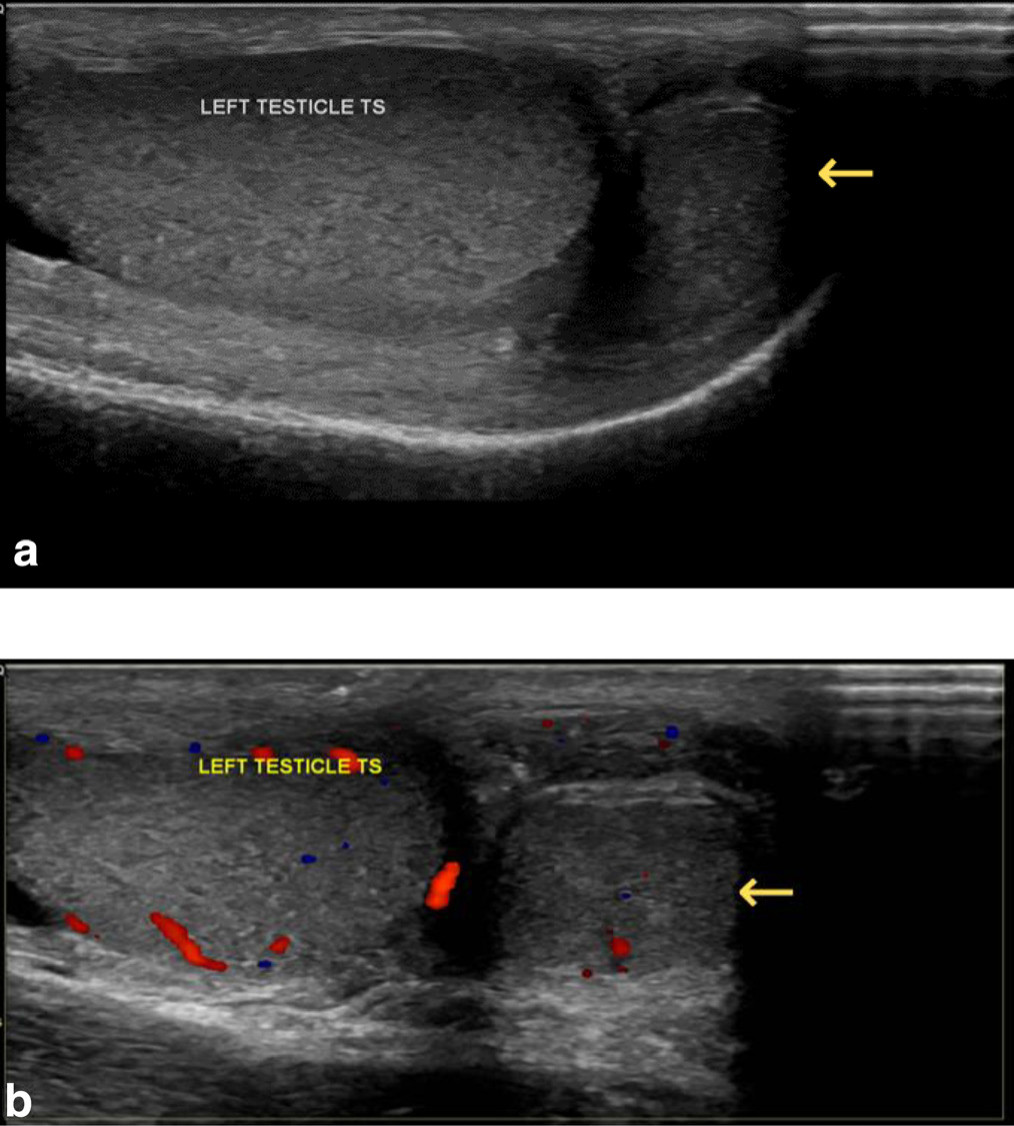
Longitudinal US image (A) and colour doppler US image (B) of the left testis showing a soft tissue mass adjacent to the left testis with similar sonographic appearances.
